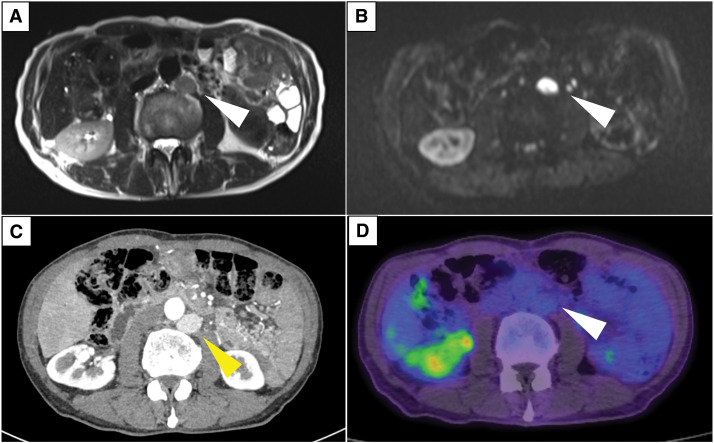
Imaging findings when PALN enlargement was detected. MRI revealed a 16-mm PALN enlargement indicated by white arrowheads. (A) T2-weighted image showing a hyperintense PALN. (B) Diffusion-weighted image demonstrating restricted diffusion in the same lesion. (C) Contrast-enhanced CT demonstrating the same lesion, indicated by a yellow arrowhead. (D) FDG PET-CT demonstrated no significant abnormal uptake in the enlarged lymph node, indicated by a white arrowhead. FDG, fluorodeoxyglucose; PALN, para-aortic lymph node
